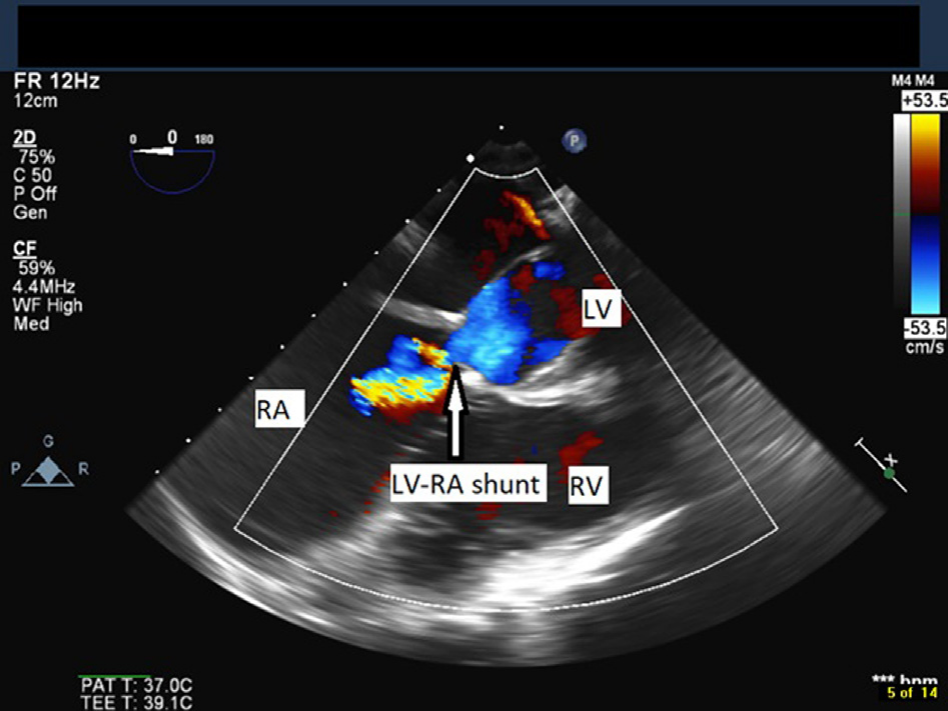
Two-dimensional TEE, midesophageal modified 4-chamber view (0°) with color flow Doppler, systolic phase, demonstrates the LV-RA shunt. *RV*, Right ventricle.
